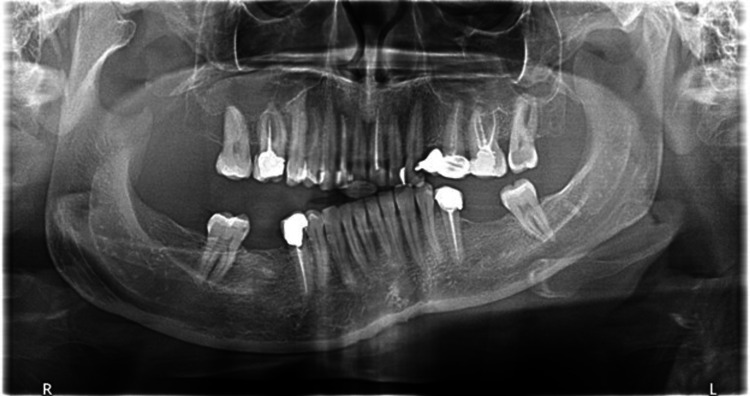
Panoramic radiograph of the mandible demonstrating an asymmetric enlargement of the right mandibular condyle and elongation of the right mandibular neck. Dental malocclusion on the right side can be seen.
